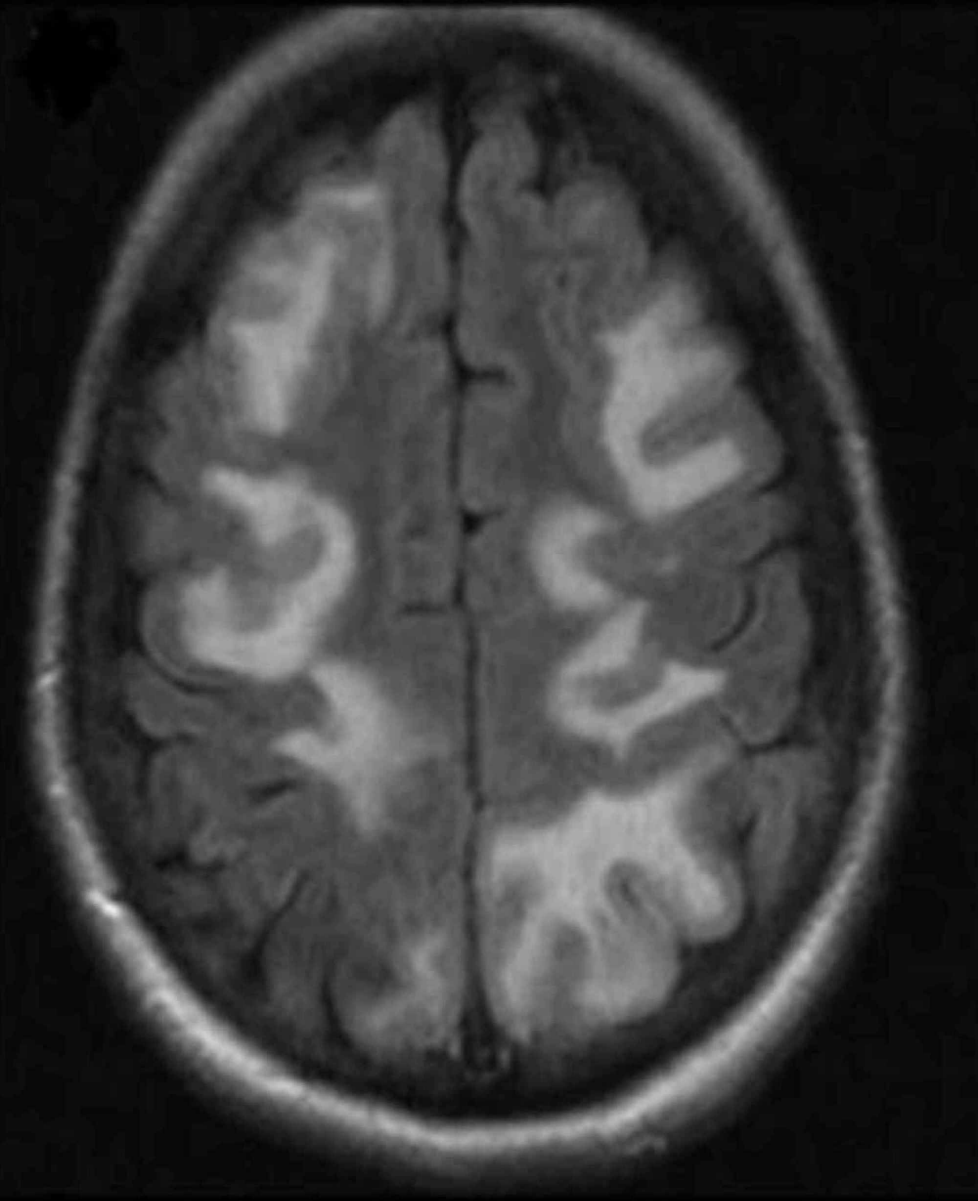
MRI findings of Posterior Reversible Encephalopathy Syndrome reproduced with permission from Wolters Kluwer Health Inc. License [3]
